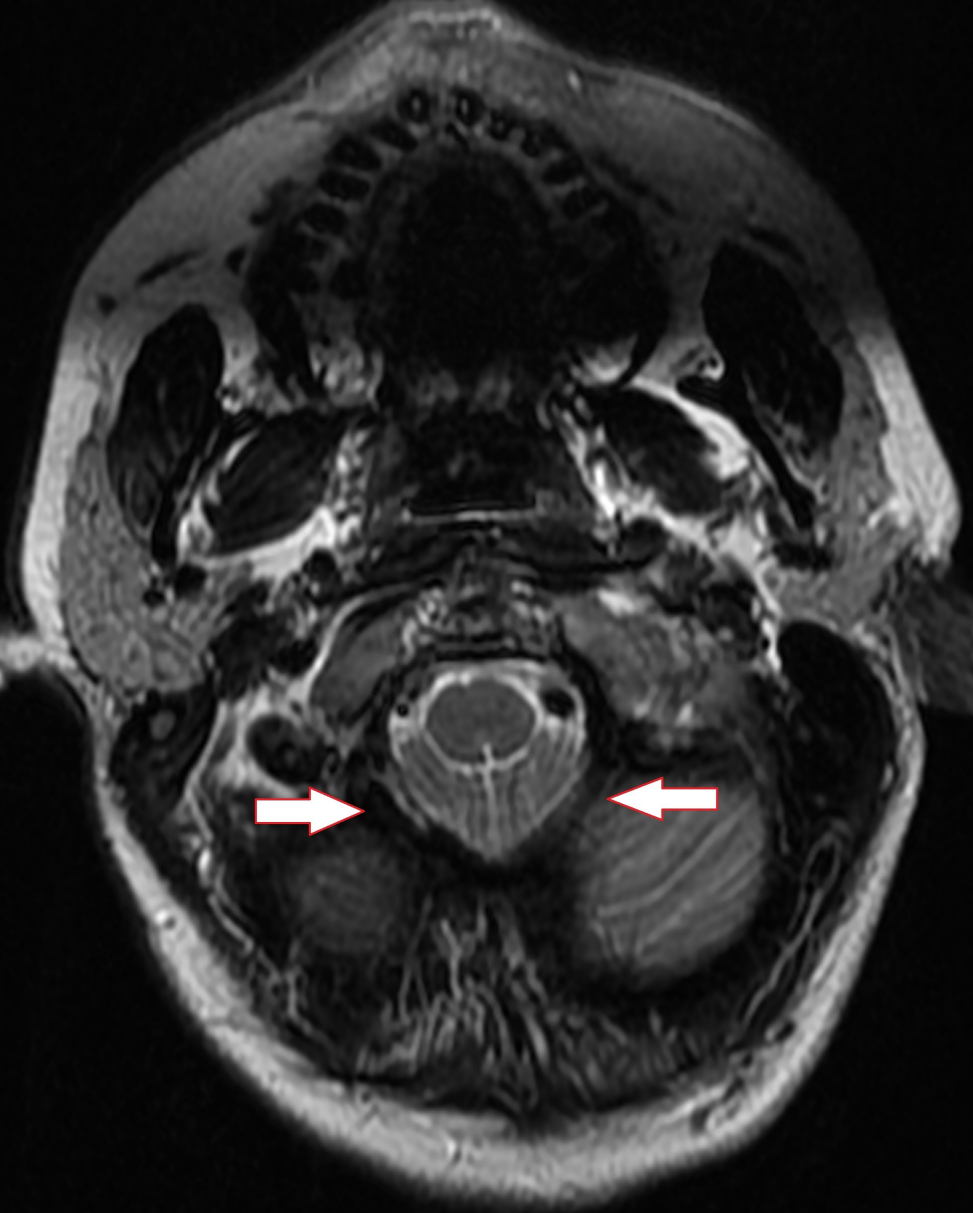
Axial T2 cervical spine MRI at the skull base demonstrating crowding of the foramen magnum and inferior descent of the cerebellar tonsils
